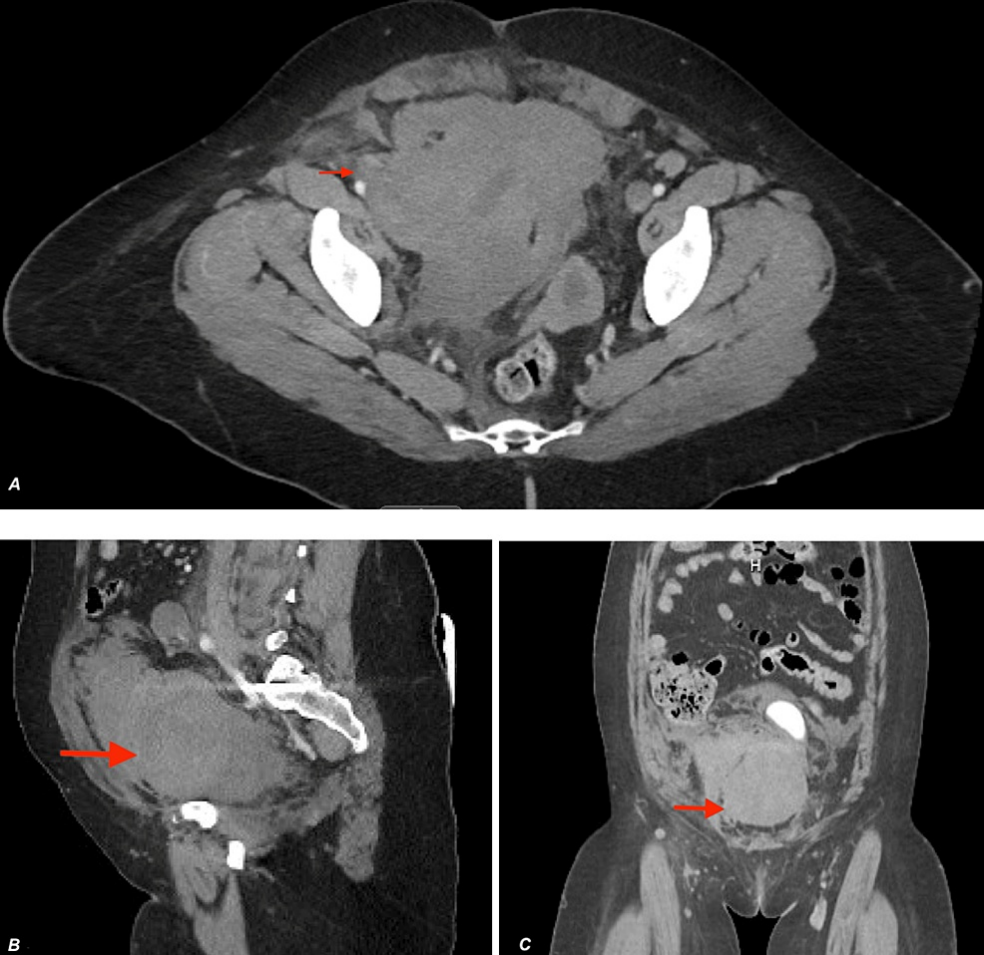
Axial (A), sagittal (B), and coronal (C) CT angiography images of the lower extremity demonstrating hematoma. CT, computed tomography
